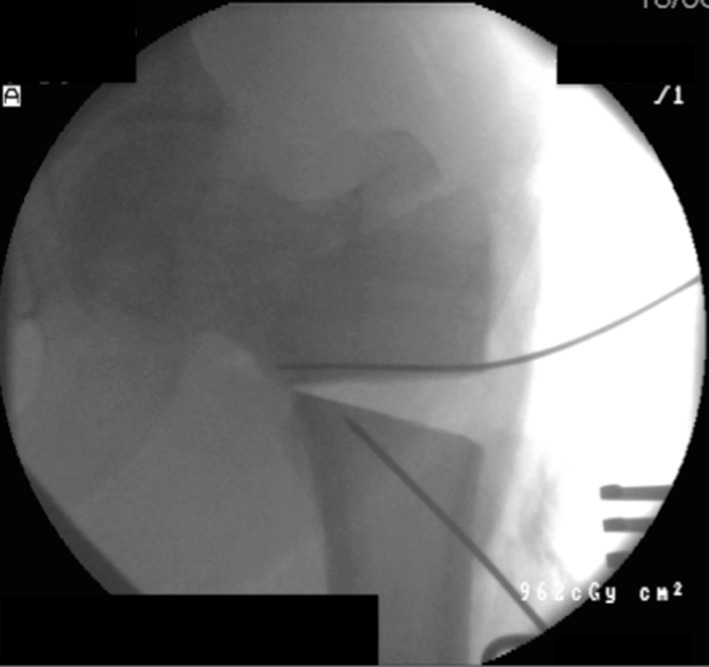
This radiograph demonstrates a proximal femoral Pauwels osteotomy, which was first introduced in 1927. It is an osteotomy, which reorientates shear forces to compressive forces. This is essentially a closing wedge osteotomy
